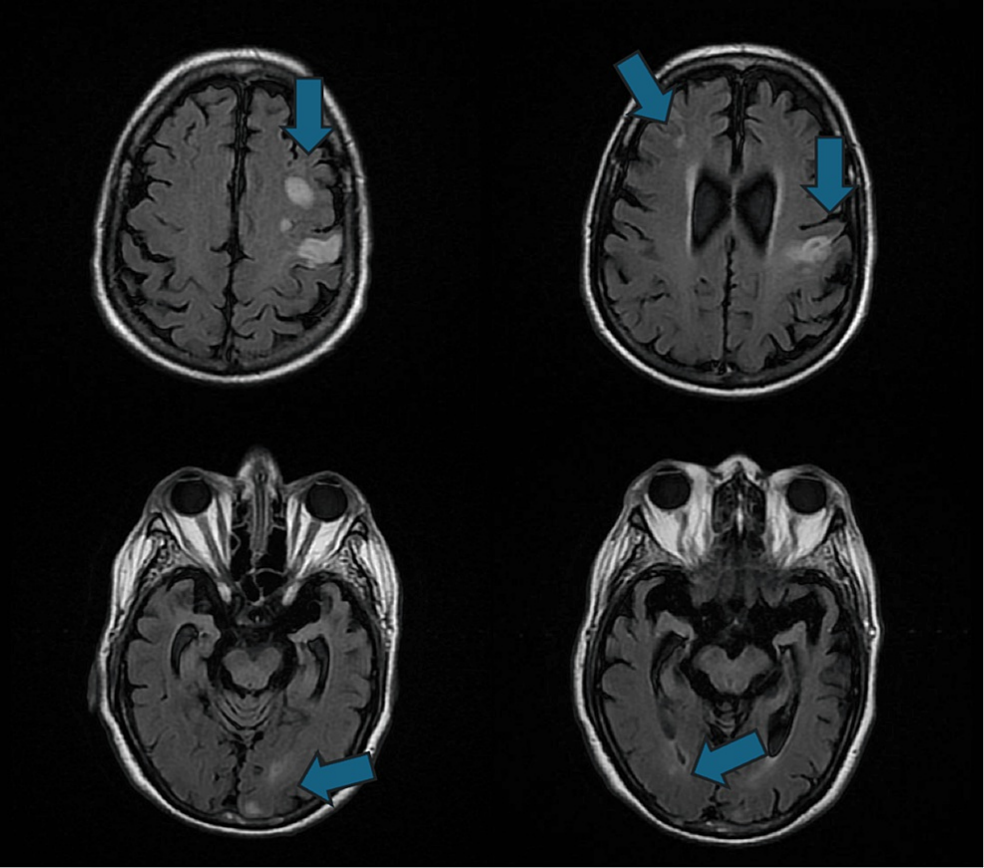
Magnetic resonance imaging of the brain. Axial T2 fluid attenuated inversion recovery (FLAIR) imaging
